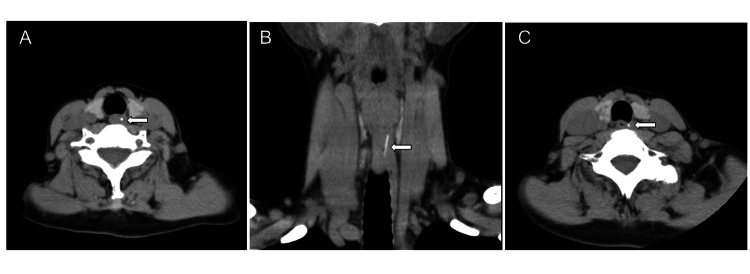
(A) Axial CT of the neck showing the fish bone (arrow) in the esophagus. (B) Coronal CT of the neck showing the fish bone (arrow) positioned obliquely downward. (C) A neck CT scan taken 19 days later showing the fish bone in nearly the same position as the previous images.
